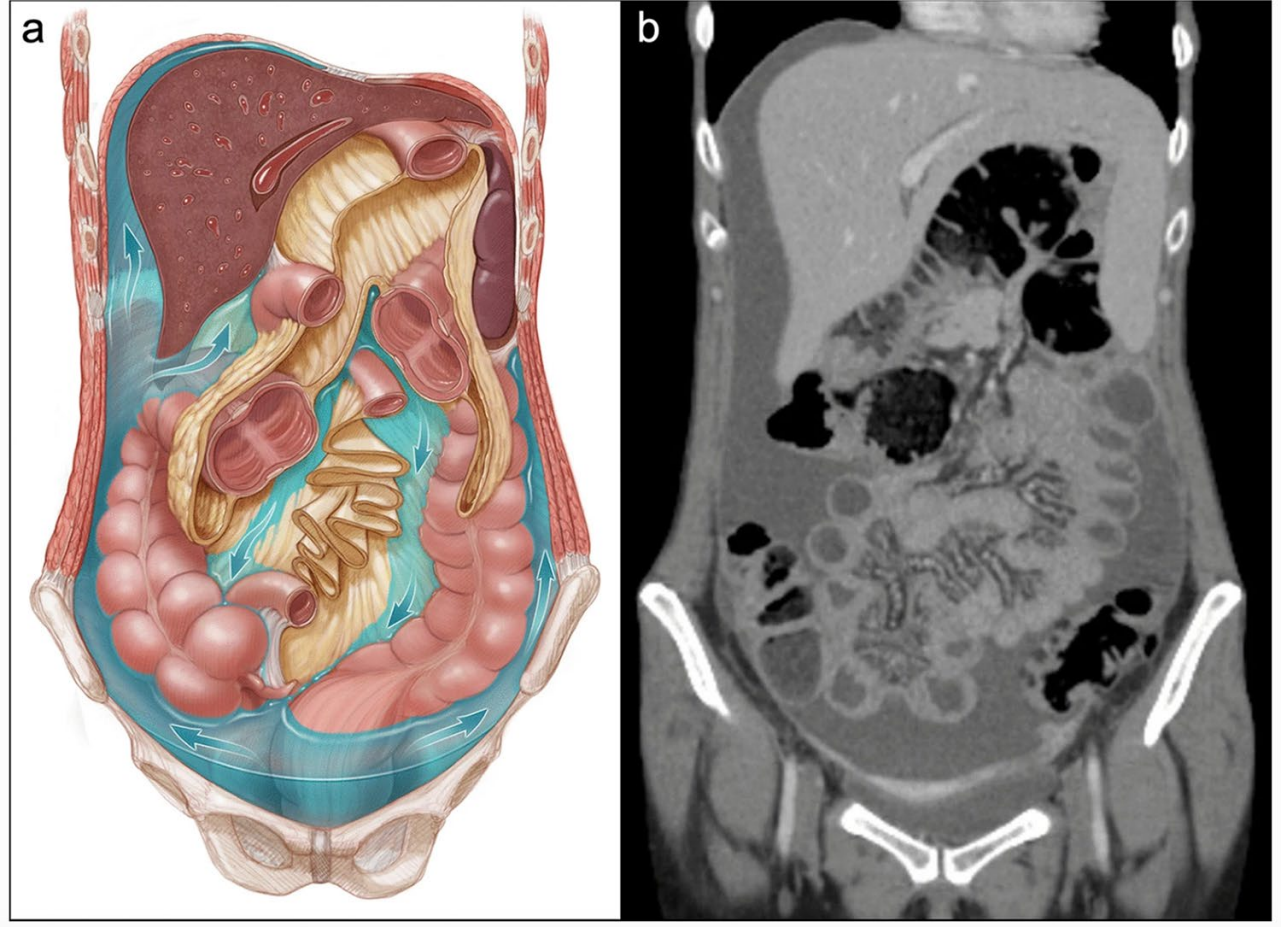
Flow dynamics of ascitic fluid in the peritoneum. A In the abdominal cavity, ascitic fluid initially collects in the pelvic recesses due to gravity. Accumulated fluids then flow upwards, pushed by the pressure gradient toward the Morison pouch and the right subphrenic space, along the right paracolic gutter. Ascitic fluid also flows upwards along the left paracolic gutter, albeit the phrenicocolic ligament limits this. B Coronal CT image depicting PM secondary to metastatic gastric cancer, with ascites in the pelvis, right paracolic gutter and right subphrenic space. Reproduced from Gonzalez et al. with permission [155]
